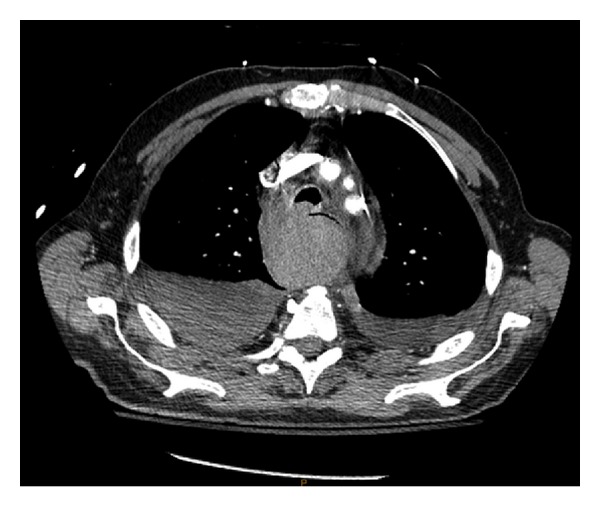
Posterior Mediastinal Hematoma measuring 5.9 cm × 5.8 cm × 21 cm, identified via CT scan upon patient presentation to MGH.
